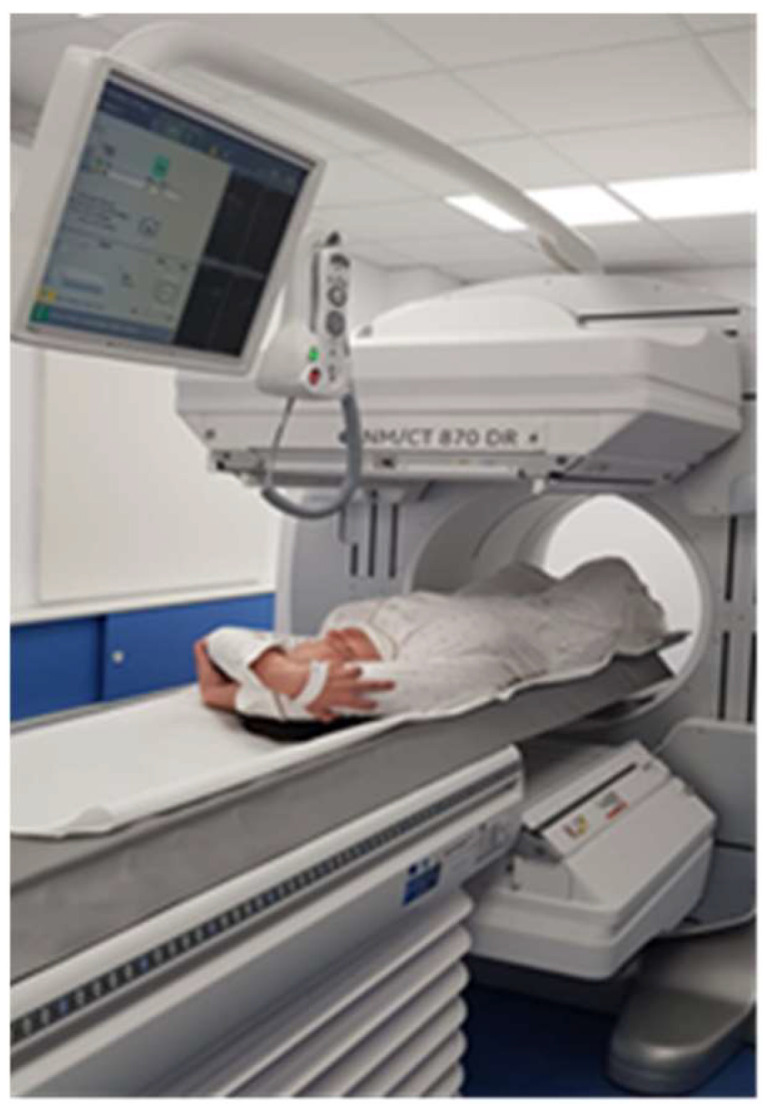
General Electric OPTIMA NM/CT 870DR SPECT-CT gamma camera which precisely locates (due to X-ray low-dose computed tomographic images) the functional abnormalities visualized in scintigraphy. Nuclear Medicine Laboratory, “Sfântul Spiridon” Clinic County Emergency Hospital, Iași.
